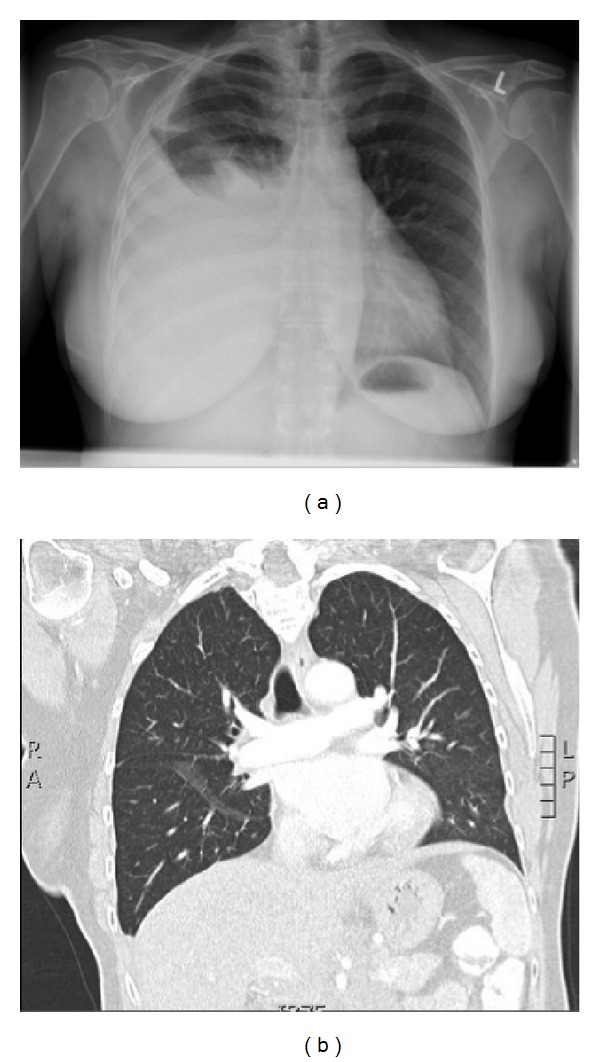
Chest X-ray before radiotherapy: large amount of pleural fluid (right) (a) with complete remission 3 months after radiotherapy on CT thorax (b).
